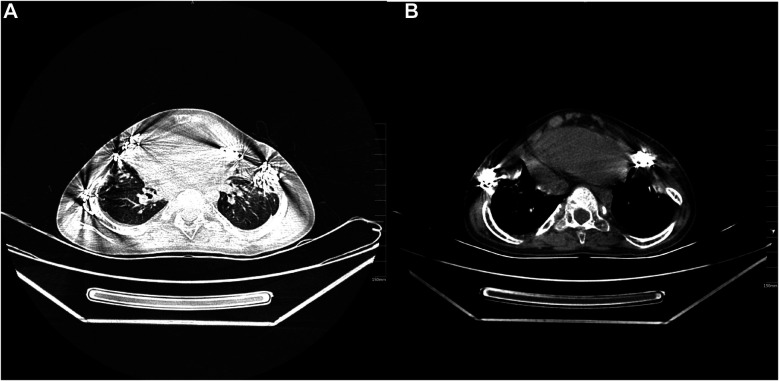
Follow-up chest CT imaging observation one and a half months after discharge. (A) The axial CT images demonstrate improved chest wall deformity following corrective surgery and the initial recovery of postoperative pulmonary exudation and inflammatory response. (B) The enhanced axial CT images provide a more precise visualization of pulmonary exudation and structural details, revealing further recovery and improvement in the post-surgical period.
